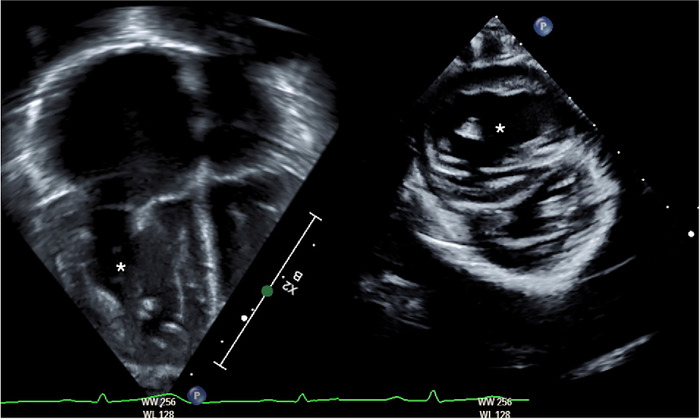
Transthoracic echocardiogram, apical 4-chamber view, shows a markedly dilated and hypertrophied right ventricle. The asterisks (*) denote the right ventricle.
